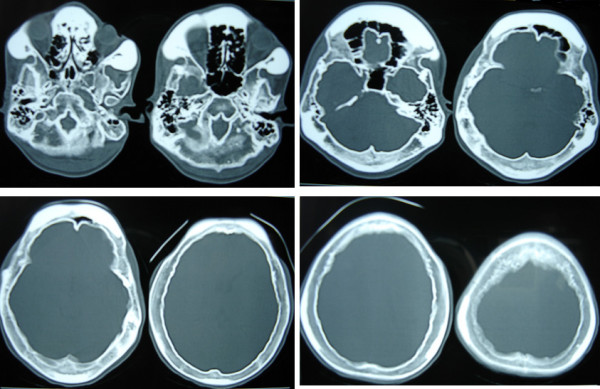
CT scan bone windows showing diffuse thickening of the skull bones with widening of the diploic space.
